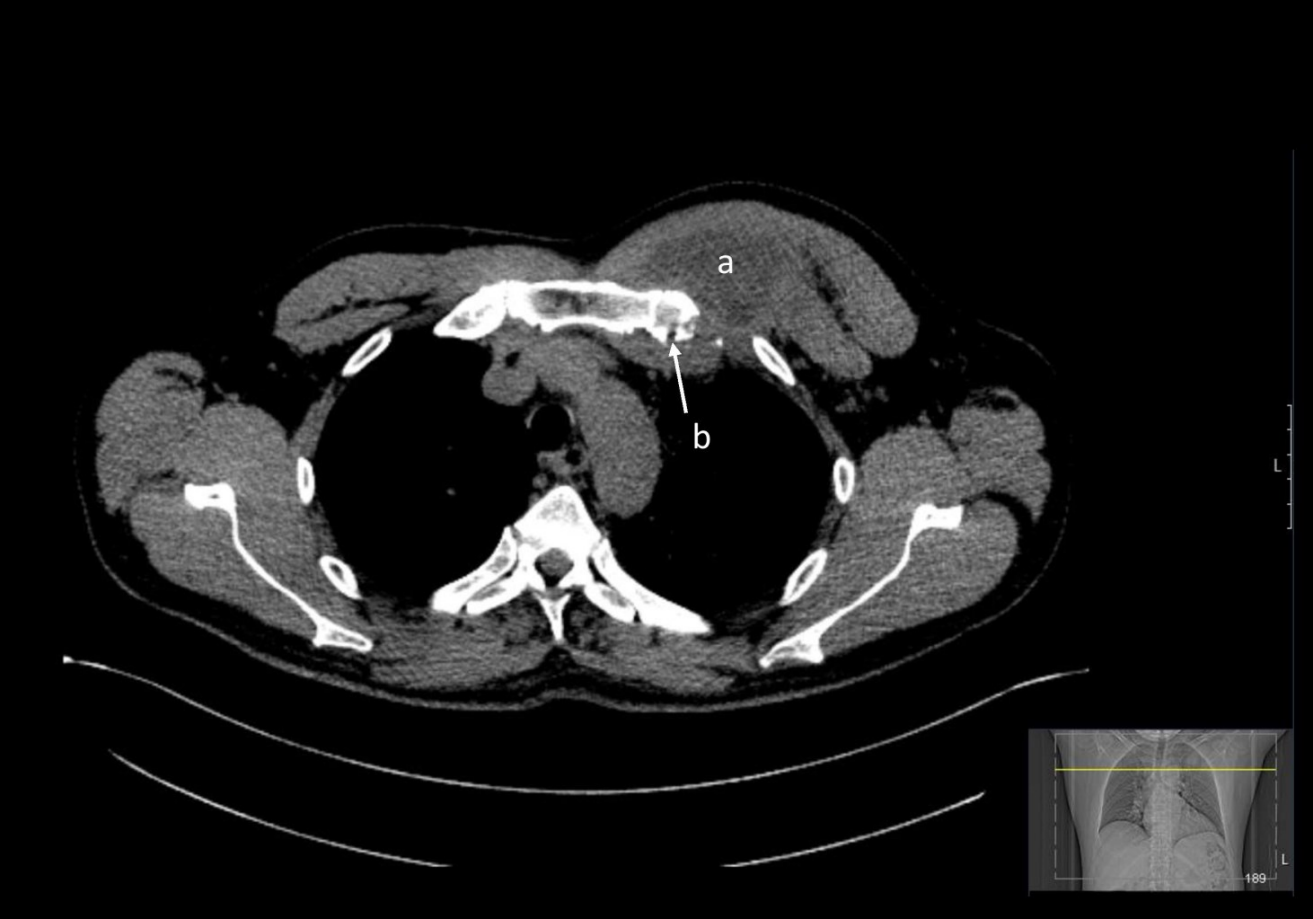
Axial view of the plain CT thorax showing low density collection in the pectoralis major muscle of the left hemithorax (a) with underlying costochondral junction showing break in cortex (b).
